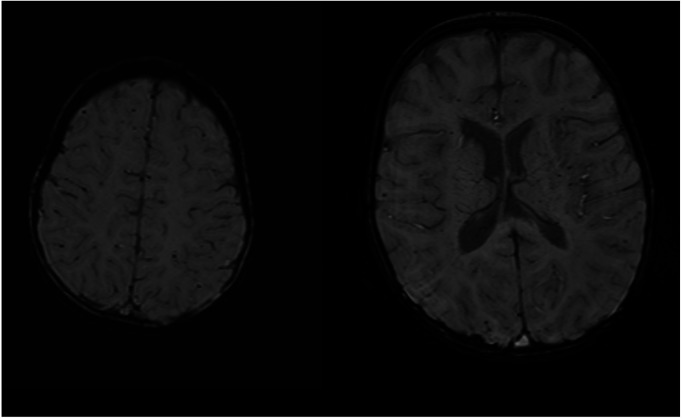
MRI screening with micro hemorrhagic foci in two patients without NDD.
